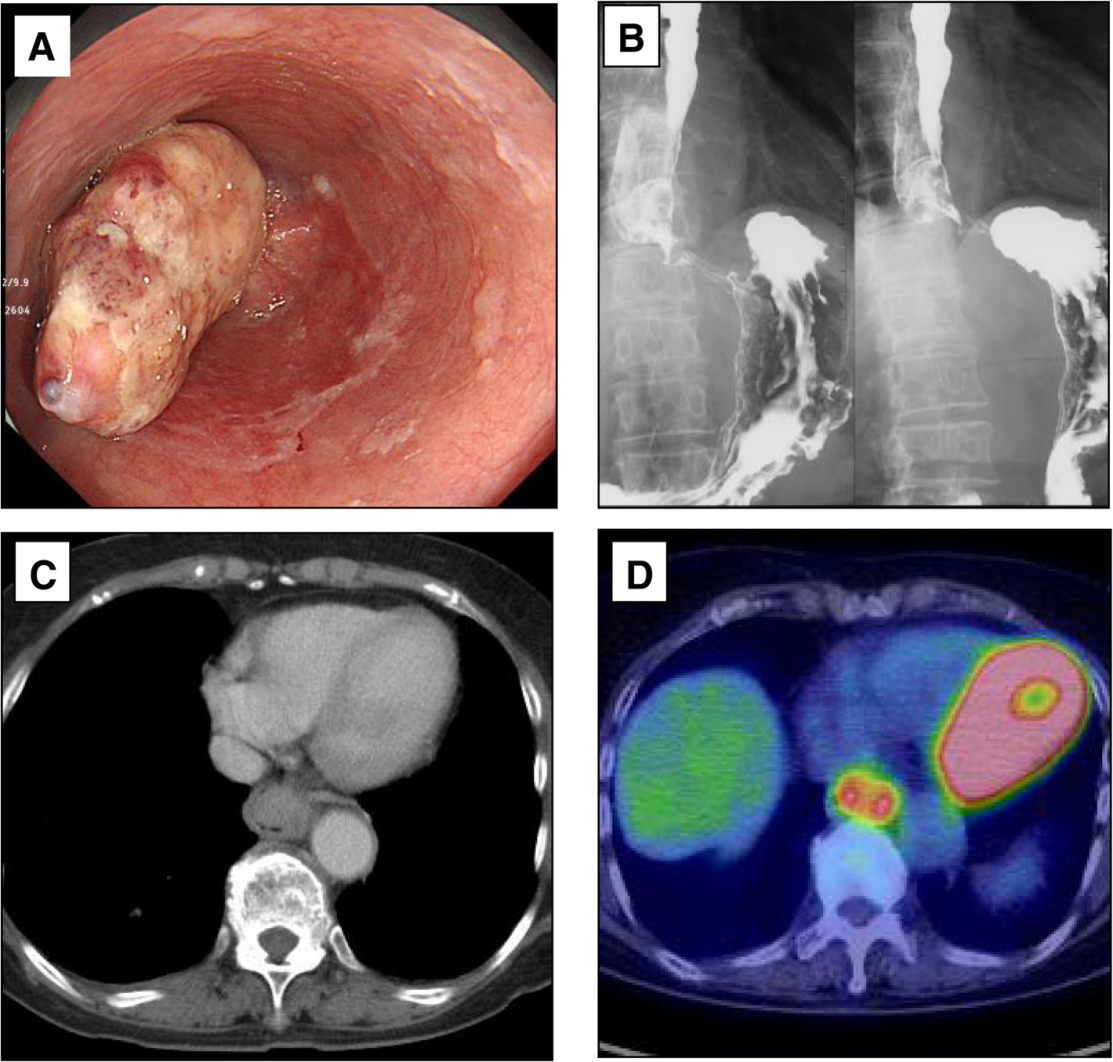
a Upper gastrointestinal endoscopy showed a type 1 tumor in the lower thoracic esophagus. b Constructed radiography showed the main tumor lesion on the left antero-lateral esophageal wall. c Contrast-enhanced CT showed that this tumor lesion measured 3 cm and occupied the esophageal lumen. d Positron emission tomography CT showed an uptake in the lower thoracic esophagus and no findings of lymph metastasis
